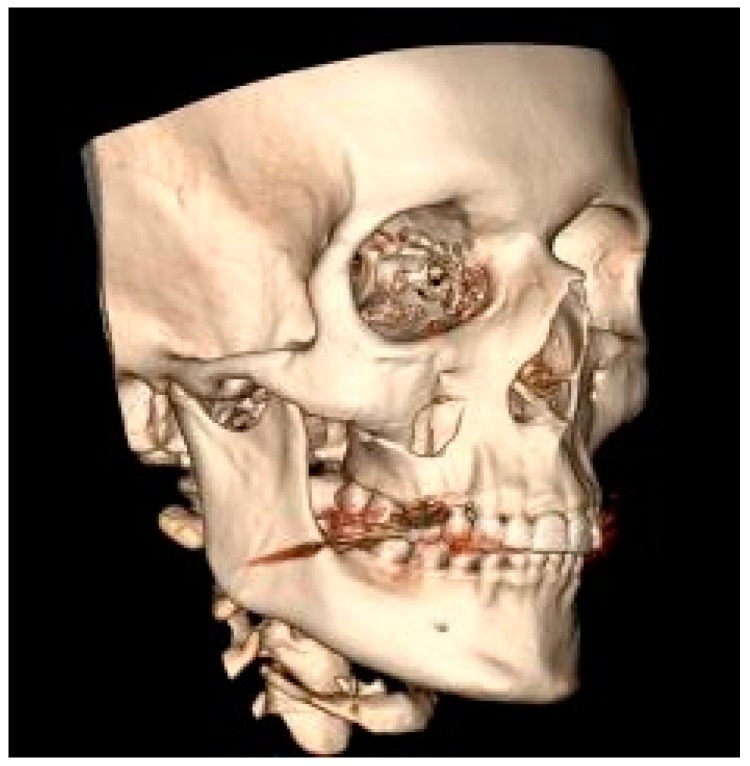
Fracture of the right maxilla (computed tomography with 3D reconstruction).
